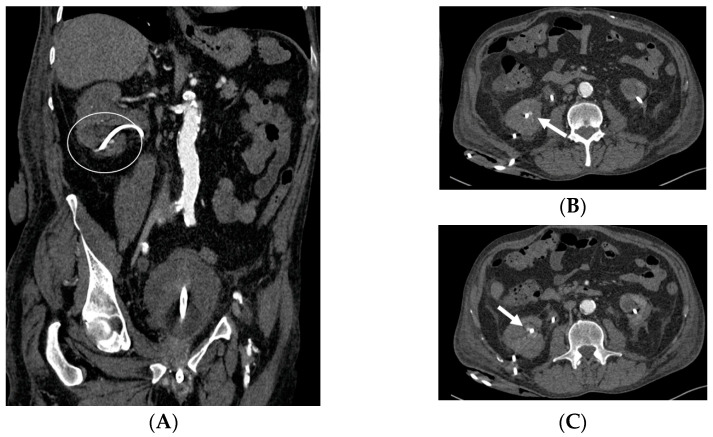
Vascular complication after nephrostomy placement. CT arterial-phase images in the coronal (A) and axial (B,C) views demonstrate the presence of multiple pseudoaneurysms (arrows) along the nephrostomy passage (circle).
